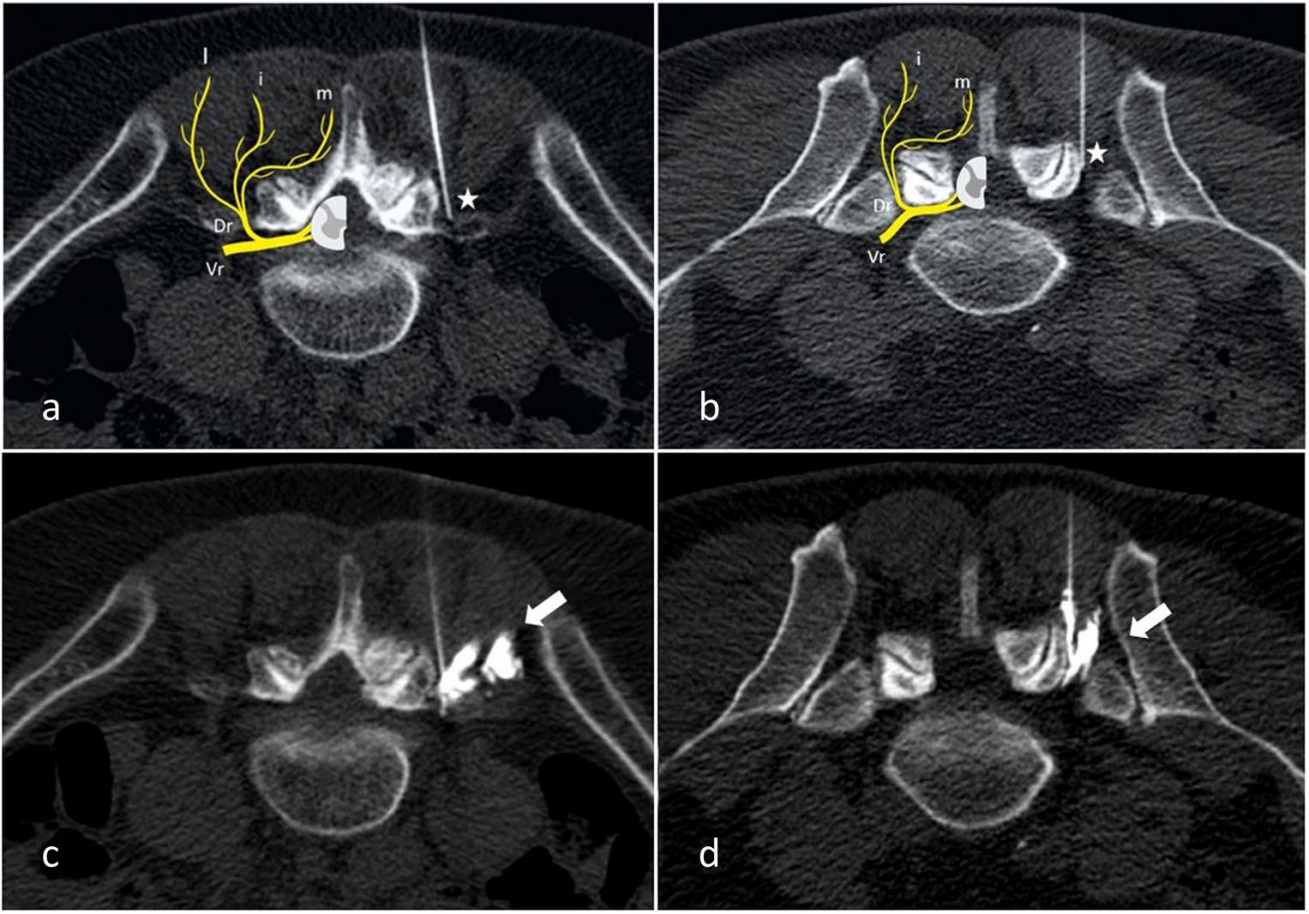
Medial branch block under CT guidance. a: L4–5 level; b: L5-S1 level. c, d: Diffusion of contrast media prior to anesthetic injection confirming optimal needle tip placement (white arrows). Needle tip at the target point at injection should be placed at the middle of the base of the transverse process at its junction with the superior process at the L4–5 level. An analogous target point should be used at the L5-S1 level midway between the upper end and middle of the ala of the sacrum (white stars). Vr: ventral ramus. Dr: Dorsal ramus. m: medial branch. i: intermediate branch. l: lateral branch
